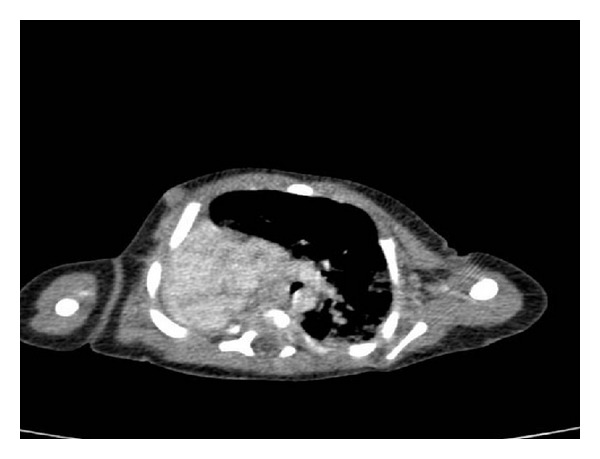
Absence of right main bronchus and right lung on CT scan.
